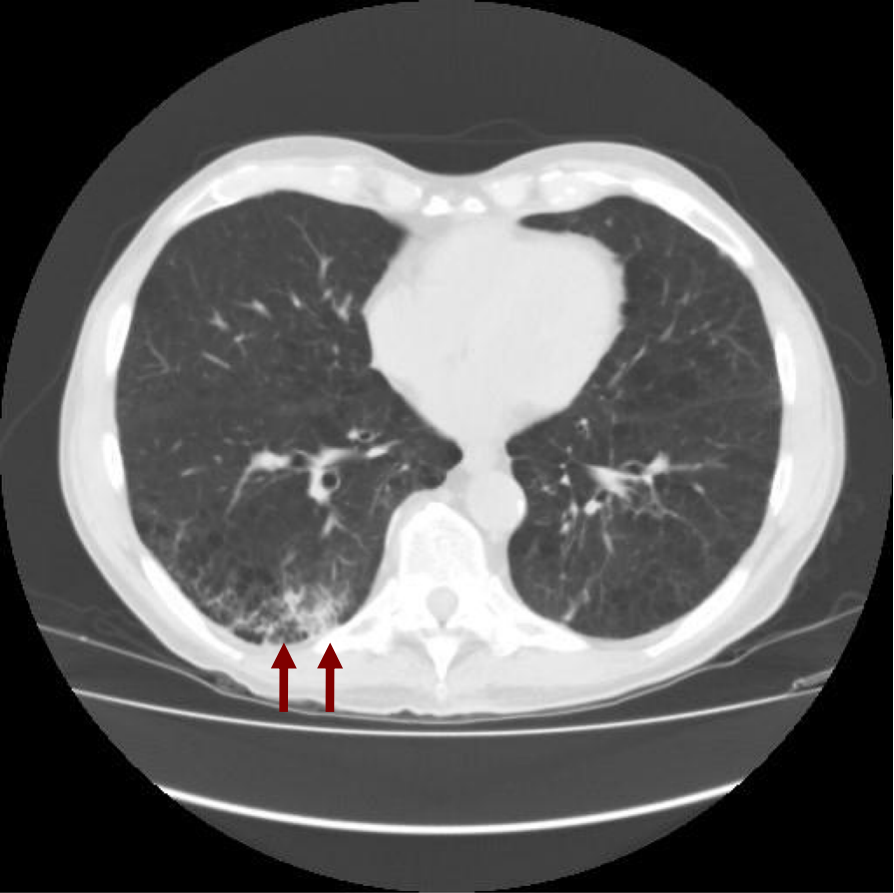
Chest CT showing lung emphysema with several pulmonary bullae and a lung infiltrate at the right lower lobe (red arrows)
